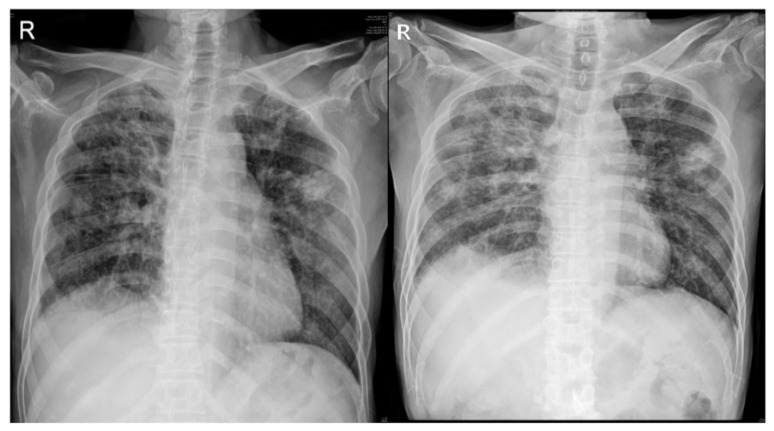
Finding on chest X-ray in the patient with COVID-19. In the first AP and second PA images, it demonstrates diffuse ground-glass opacity and a large nodular lesion in the left upper lobe.
